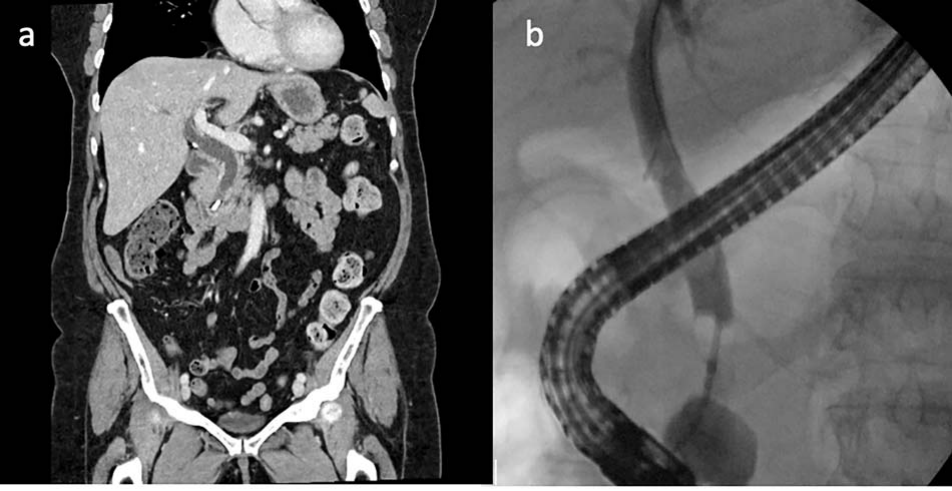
CT abdomen and ERCP. (a) CT abdomen showed a linear high density focus consistent with a 10-mm surgical clip obstructing the distal CBD, which was significantly dilated proximally (coronal). (b) ERCP showing filling defect in CBD.
